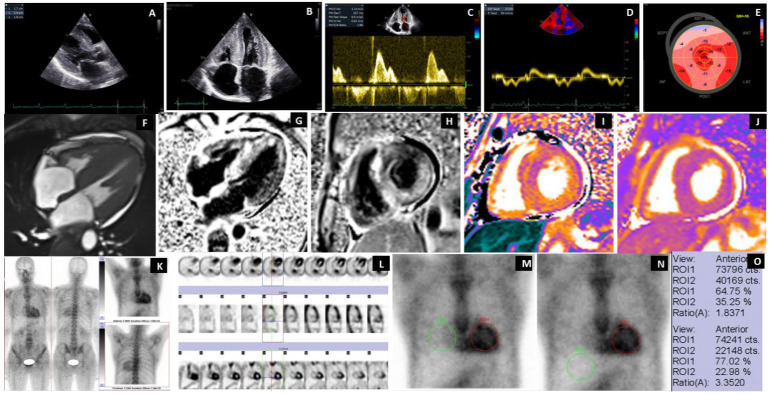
Representative case of G3 uptake in an ATTRGlu54Gln CA patient with mixed phenotype. Transthoracic echocardiography (A–E). Parasternal long axis diastolic frame (A) and apical 4 chambers systolic frame (B) of a very typical, advanced patient showing severe concentric LV hypertrophy, RV free wall hypertrophy, dilated atria, thickened atrioventricular valves, and a trace of pericardial effusion. Pulsed wave Doppler with the sample volume located at the tip of the mitral leaflets (C) and Tissue Doppler echocardiography with the sample volume located at the base of the septal mitral annulus (D), revealing advanced grade II diastolic dysfunction with an E wave which is taller than the A wave and severe longitudinal impairment of both systolic and diastolic myocardial velocities and an E/e’ of 23. Automated function imaging derived from the speckle tracking echocardiography (E) revealed a severely impaired GLS with the apical sparing pattern of the Bull’s eye view. Contrast-enhanced cardiac magnetic resonance (F–J). Cine (balanced SSFP) 4-chamber sequences (F) revealed the same findings as the transthoracic echocardiography. Late gadolinium enhancement sequences in 4 chambers and basal short axis show diffuse, transmural LGE, which also involves the papillary muscles, the RV-free wall, and the atrial walls with abnormal gadolinium kinetics (G,H). Noncontrast T1 mapping acquired on a 1.5 T scanner revealed a severely elevated native T1 of 1265 ms, corresponding to a severe extracellular expansion (ECV 63%) (I). A slightly increased native T2 (56 ms) reflecting a degree of myocardial edema (J). Bisphosphonate scintigraphy (K–O). Planar Whole-Body acquisition in the anterior and posterior view from the vertex to the proximal 1/3 of the thighs. Static acquisition at the chest in the anterior and posterior views. Diffusely increased uptake was observed at the heart, with intensity greater than the costal one, with a Perugini visual uptake score of G3 (K). Acquisition of SPECT at the chest, with the delimitation of the LV walls and free wall of the RV, after the reconstruction and display of the transverse, sagittal, and coronal slices (L). Selection of ROIs from the LV level and the contralateral region where the RV overlaps (M). Selection of ROIs at the level of the LV and the right subdiaphragmatic, in the liver region without including the RV (N). The difference in the semiquantitative score of the H/CL (*n* = 1.83) and H/L (*n* = 3.35) ratios is due to the overlap of the RV. (O) ECV = extracellular volume; GLS = global longitudinal strain; LGE = late gadolinium enhancement; LV = left ventricle; ROI = region of interest; RV = right ventricle; SPECT = single-photon emission computer tomography; SSFP=steady-state free precession.
